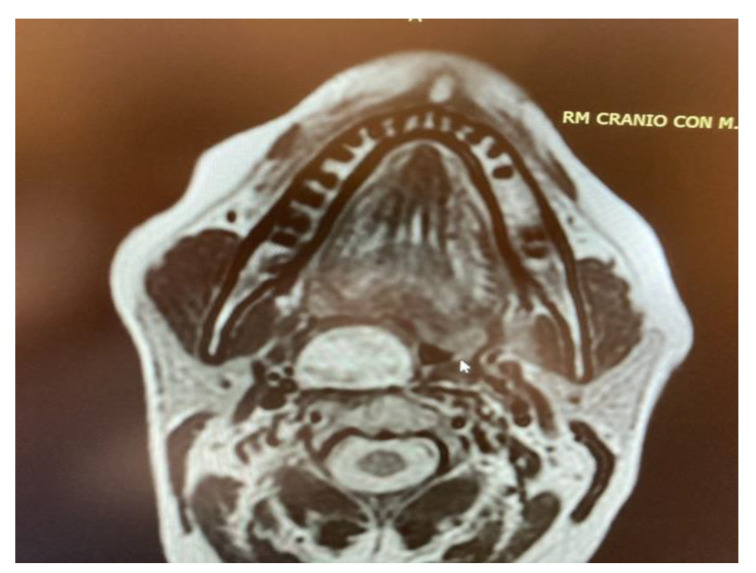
MRI T2 weighted image showing a right parapharyngeal schwannoma arising from the cervical sympathetic chain. The schwannoma does not dislocate the big vessels in the typical anterior direction, but the big vessels’ dislocation is posterolateral. However, the parapharyngeal fat pad is dislocated in an anterior direction, and this suggests a carotid space tumour.
